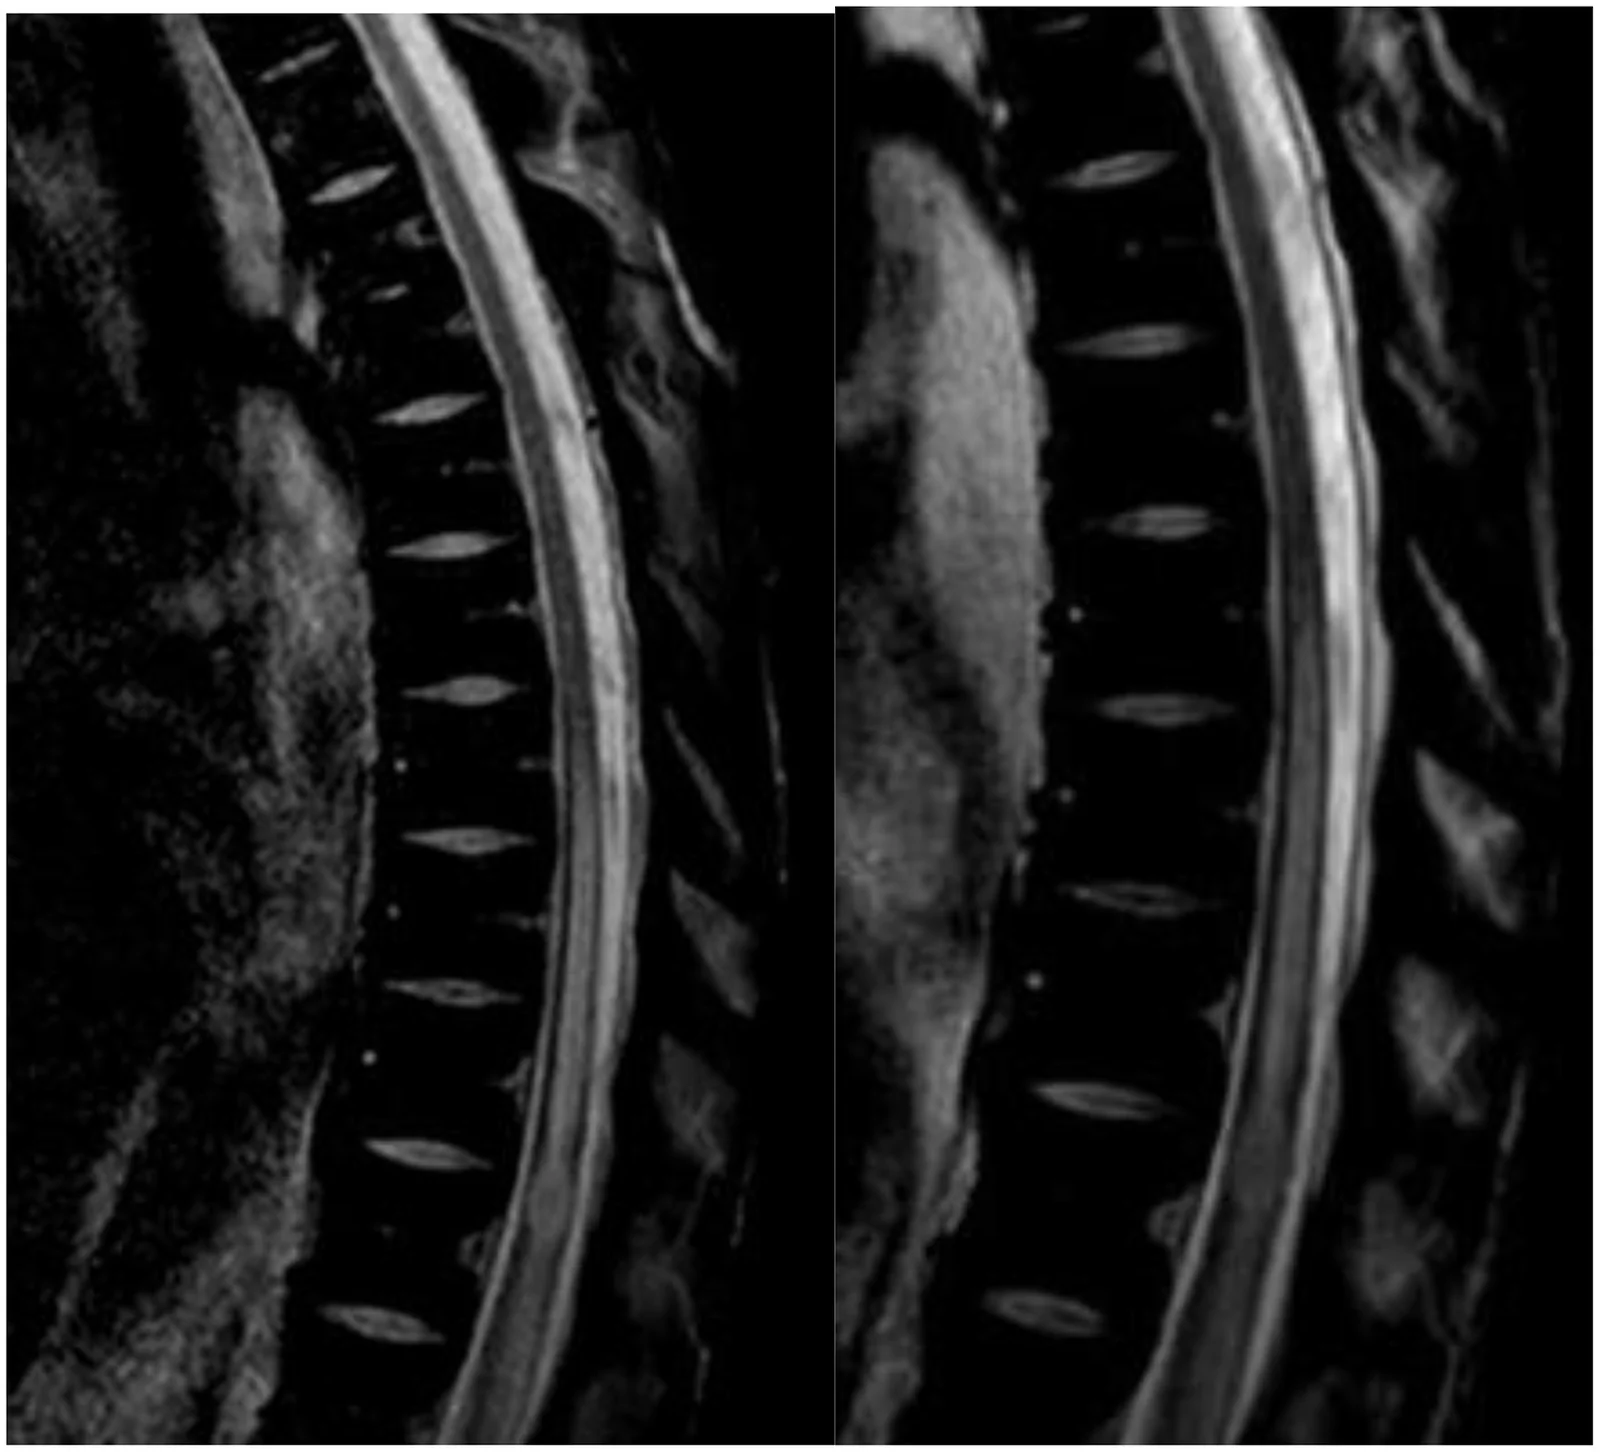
Sagittal T2-weighted MRI demonstrates a nodular intramedullary lesion at the T10-T11 level, exhibiting intermediate signal intensity. An associated extensive area of vasogenic edema is present, extending cranially from T9 to T12, resulting in significant cord involvement across multiple vertebral levels.
